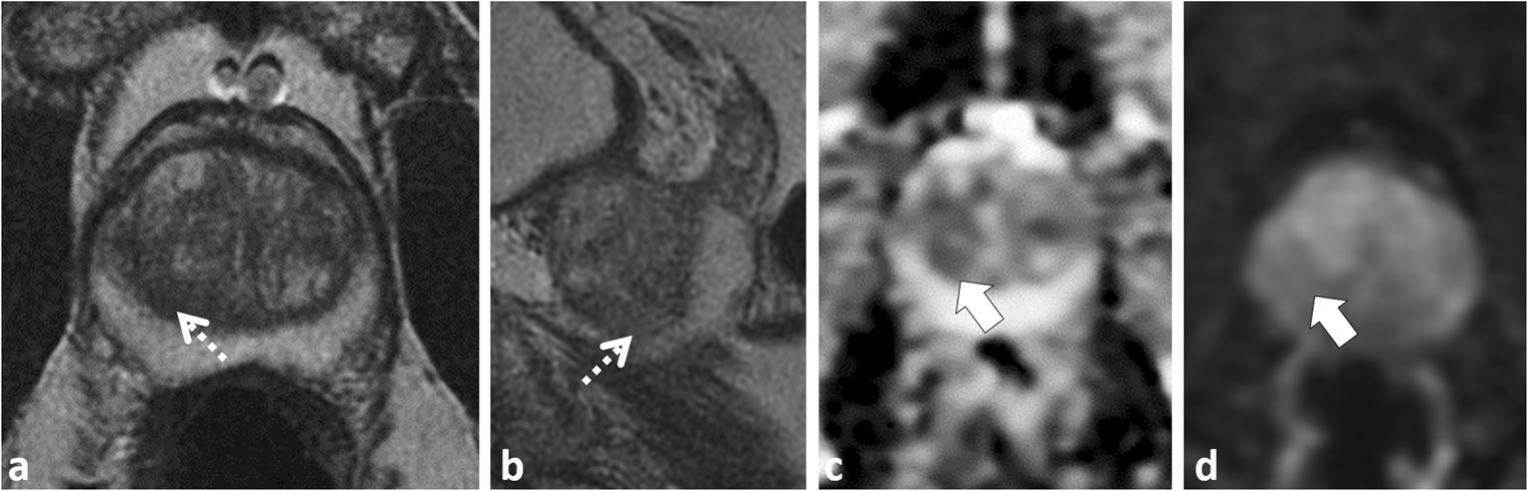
A 51-year-old patient with low-volume Gleason score 3 + 3 = 6 at non-targeted TRUS-guided biopsy. MP-MRI was performed at onset of active surveillance (AS) to exclude higher grade tumour. Axial (a) and sagittal (b) T2W images demonstrate an ill-defined ovoid lesion of low T2 SI at the junction of the right PZ and transition zone (TZ) (dotted *white arrow*) along the course of the surgical capsule. Axial ADC map image (c) at the same level demonstrates corresponding low SI (*white arrow*). This area was considered suspicious for tumour. Targeted TRUS biopsy was performed and yielded only normal prostate tissue. The area did not change on follow-up MP-MRI and represents asymmetry of the normal central zone. In retrospect, corresponding b1000 mm²/s trace EPI (d) did not show increased signal (*white arrow*) in this area
